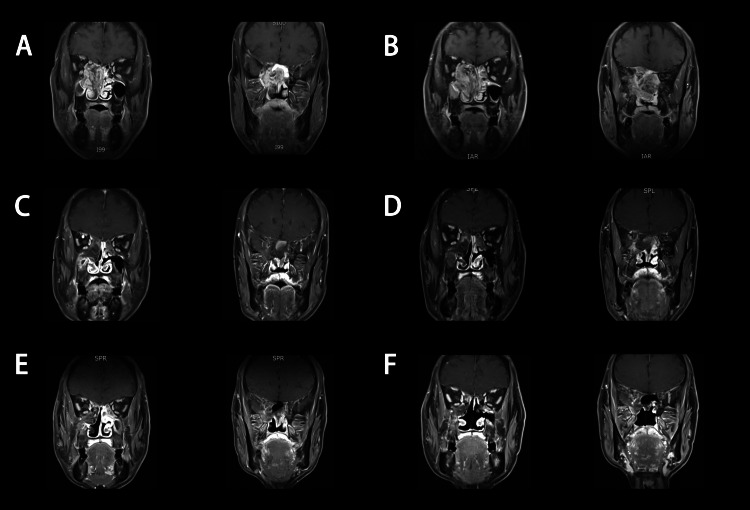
Coronal T1- weighted on the administration of contrast MR imaging At the time of diagnosis (A), 18 days after surgery (B), 1.5 months after treatment (C), three months after treatment (D), seven months after treatment (E) and 13 months after treatment (F).
